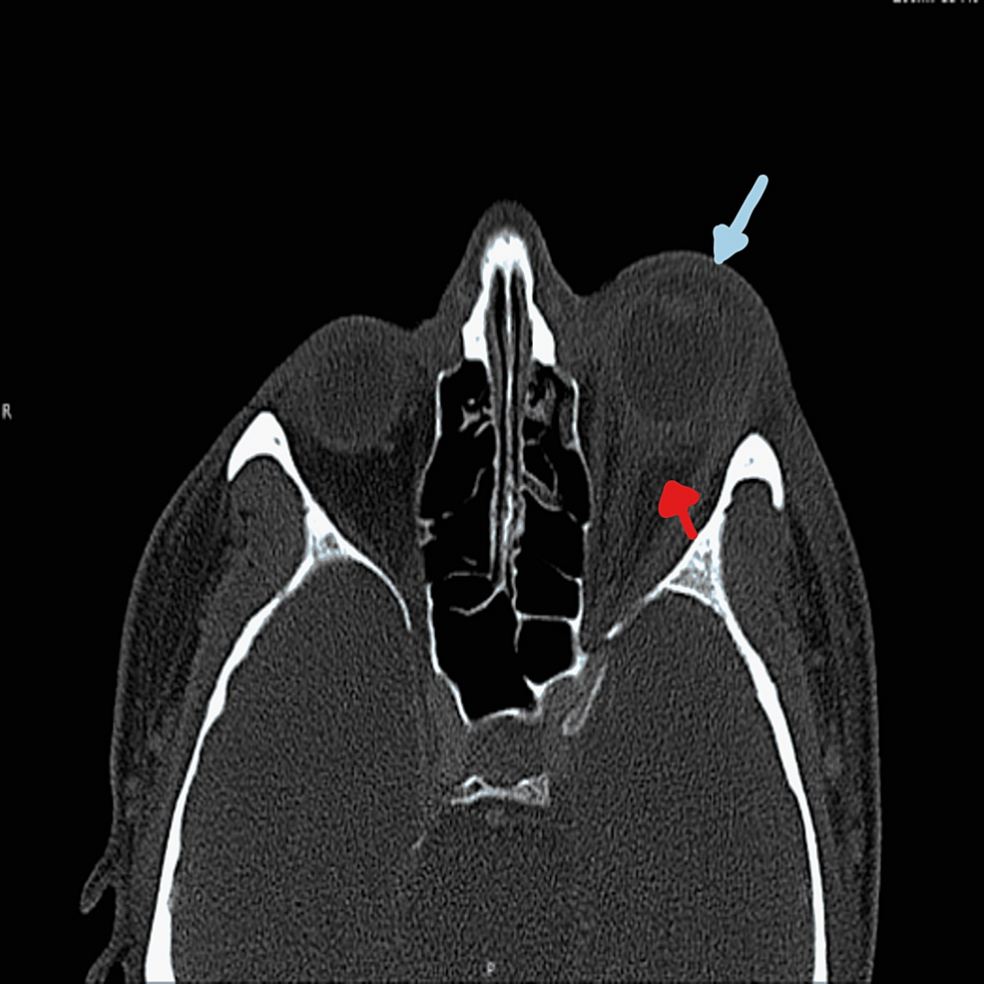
CT scan (skull) of facial bones and periorbital sinuses (blue and red arrows).
